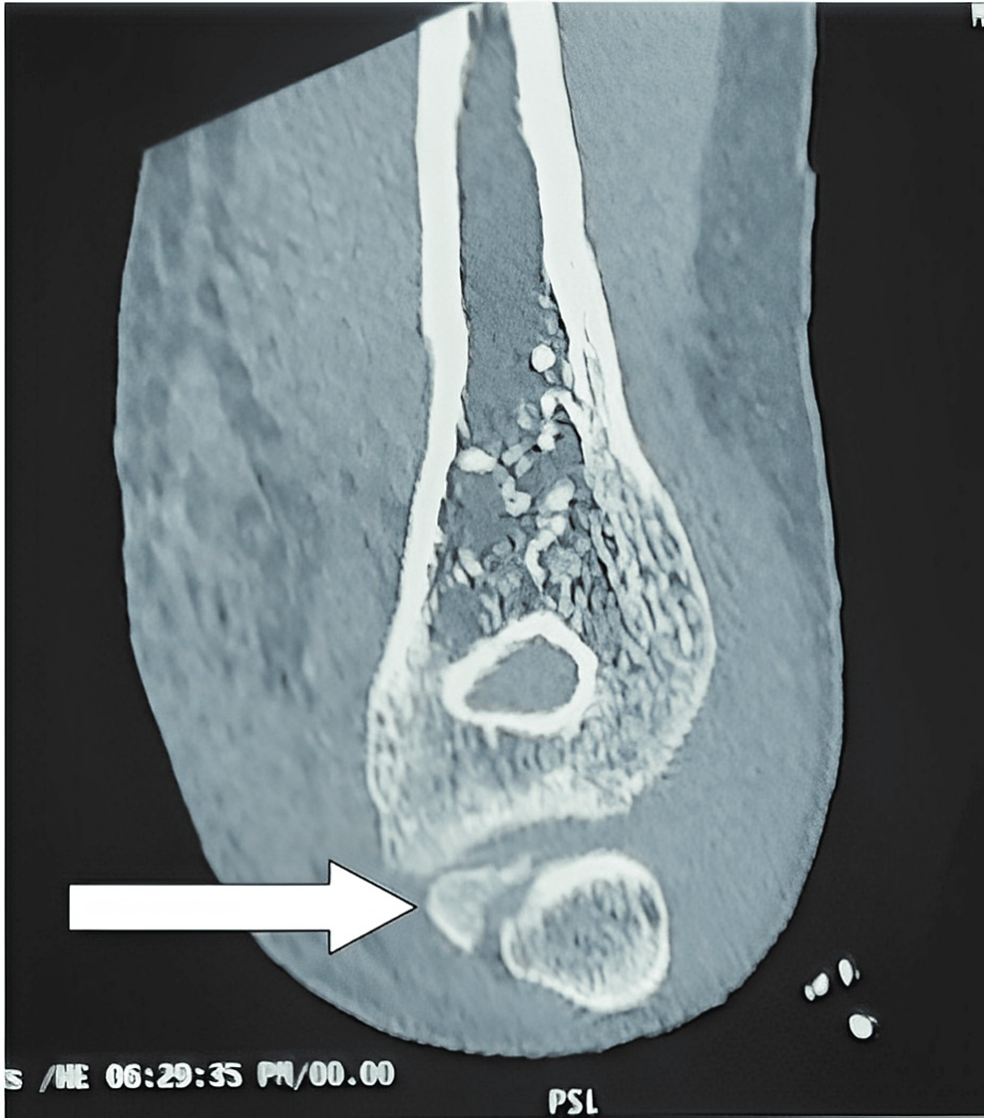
CT of left elbow (coronal image) showing the fragment (white arrow) into the joint
